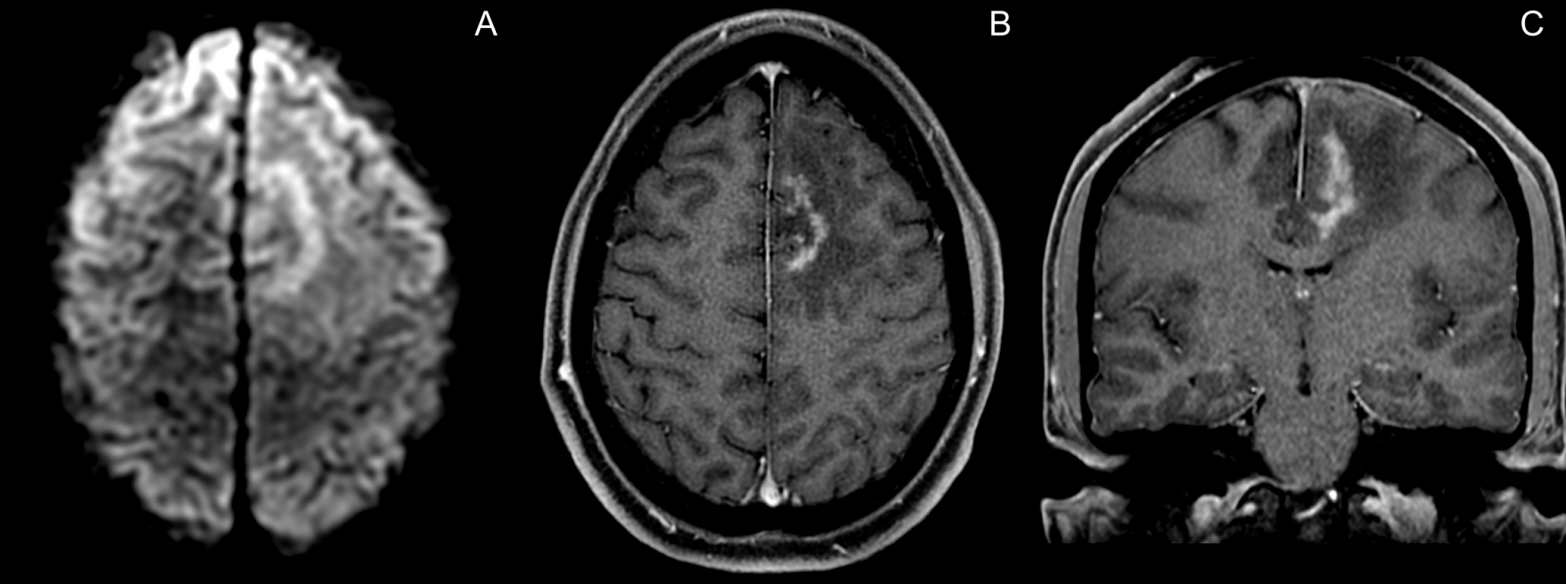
A: Axial diffusion weighted imaging (DWI), showing a left frontal lesion with restricted diffusion on the lesion’s free margin, oriented towards the white matter, suggestive of demyelinating nature. B: Axial post-gadolinium T1, showing contrast enhancement on the lesions free margin. C: Coronal post-gadolinium T1 shows the left frontal lesion, insinuating towards the corpus callosum, but with no frank signs of invasion. Notice the spared brainstem.
